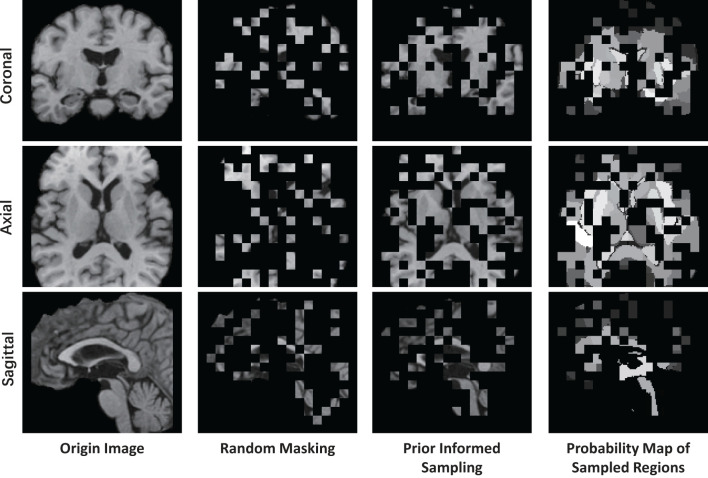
Example of visualizing a T1 MR image using different masking strategies with a masking ratio of 0.75. This image is center-cropped with a shape of 128 × 128 × 128, and each token has a patch size of 8 × 8 × 8.
